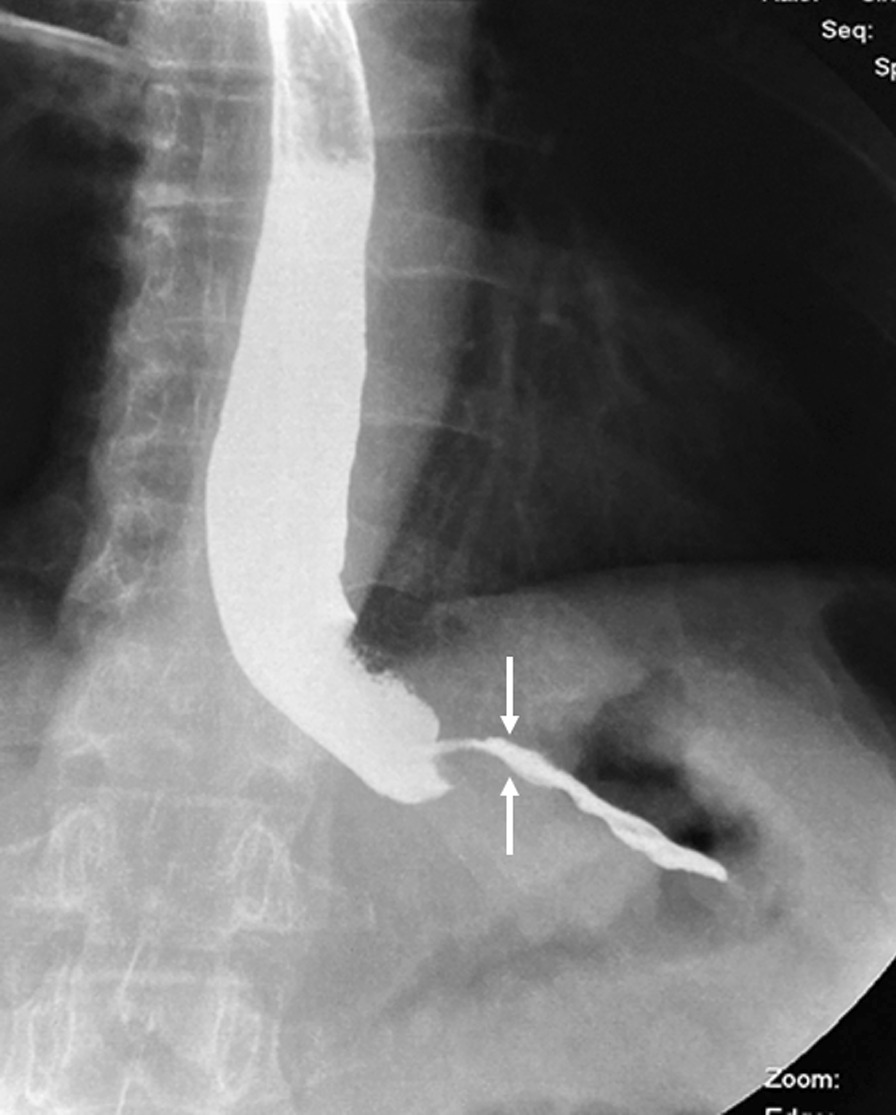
Preoperative patient’s x-ray (the arrows show the narrowed lumen of the stomach by the tumor)
